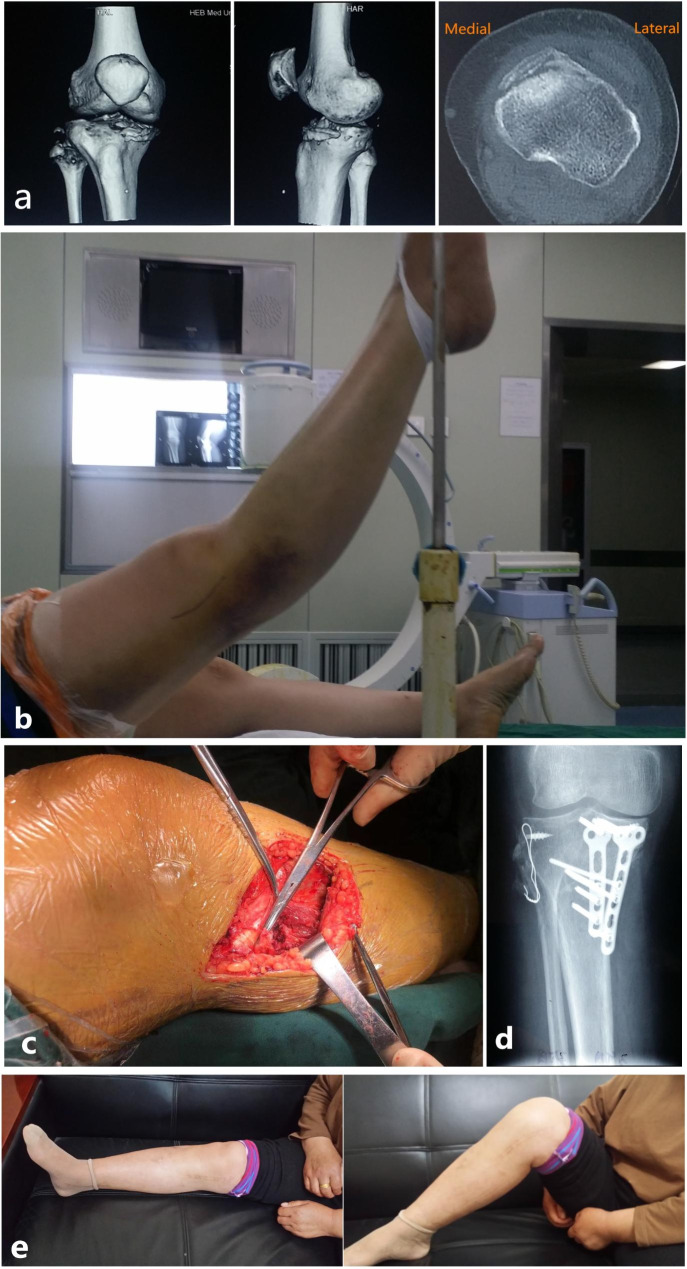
A typical case of a 42-year-old female with Schatzker IV tibial plateau fractures (hyperextension-varus-internal rotation type). (a) The features of this type were shown in CT images. (b) Preoperative hyperextension deformity suggested posterolateral instability of the knee joint. (c) The knee should be placed in flexion during the operation. (d) After restoring the compressed articular surface, two plates were needed for internal fixation. The avulsion fracture of the fibular head was fixed by rivets or wires. (e) 1 year postoperatively, the stability and function of the knee were good
